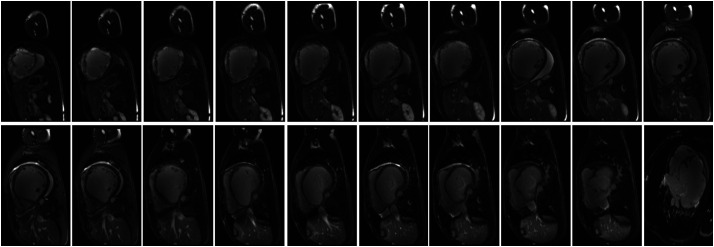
MRI of the heart showed concomitant myocardial inflammation with edema.
